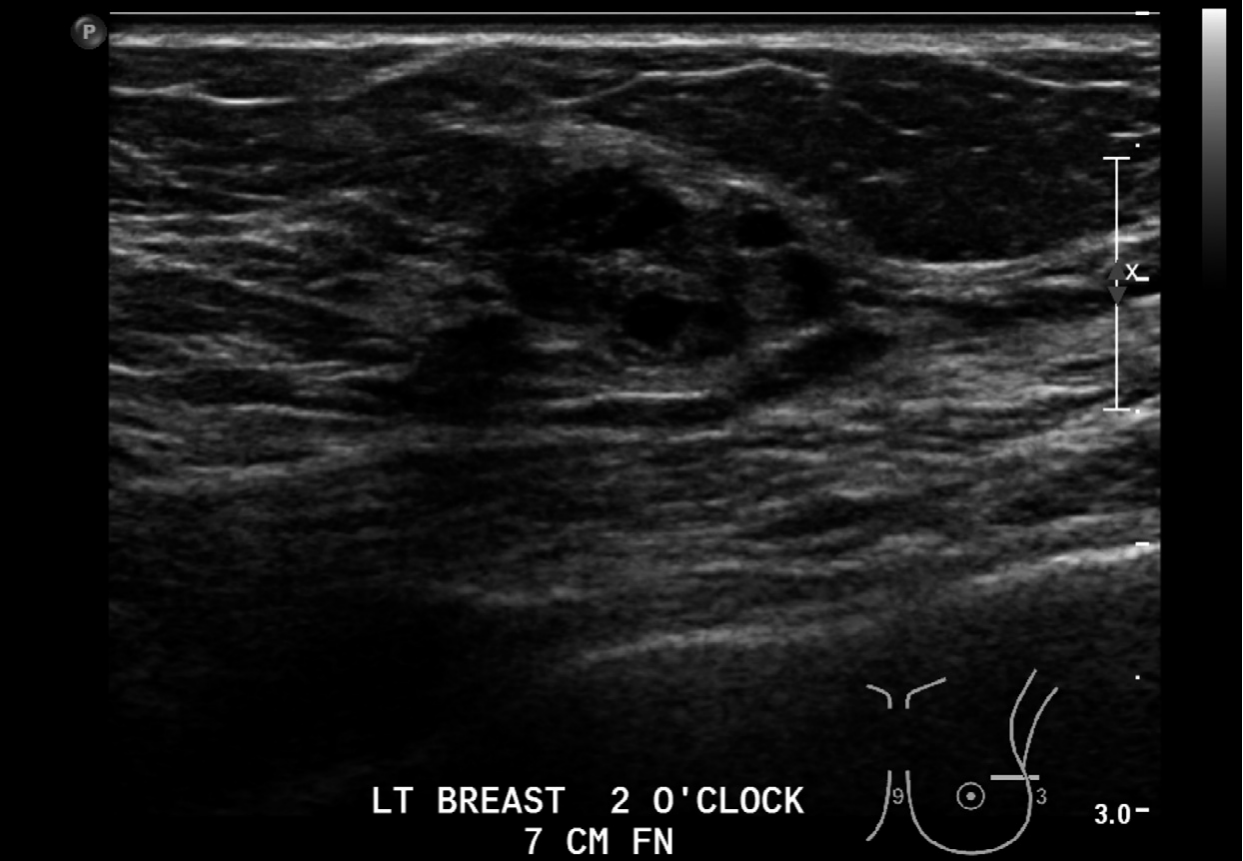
Lesion three: Ultrasound image showing an ovoid, mixed cystic and solid mass. Image courtesy of BreastScreen ACT.
